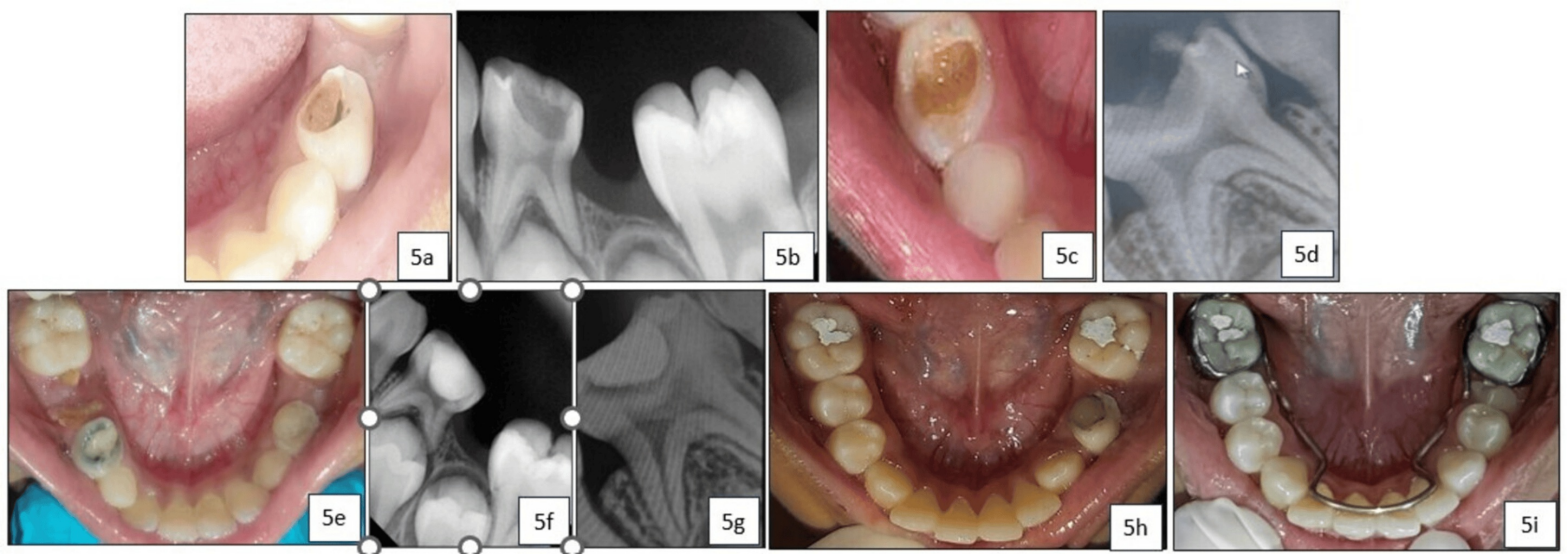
(a) Pre-operative image showing International Caries Detection and Assessment System (ICDAS) II score 5 in relation to 74; (b) Pre-operative radiograph in relation to 74; (c) Pre-operative image showing ICDAS II score 5 in relation to 84; (d) Pre-operative radiograph in relation to 84; (e) Immediate post-operative clinical view showing silver diamine fluoride + glass ionomer cement (silver-modified atraumatic restorative treatment technique) applied in relation to 74 and 84; (f) Post-operative radiograph of 74; (g) Post-operative radiograph of 84; (h) 12-month follow-up showing exfoliated 84 with erupting 44 and 45; (i) 24-month follow-up showing lingual arch space maintainer with erupting 34 and 35.
